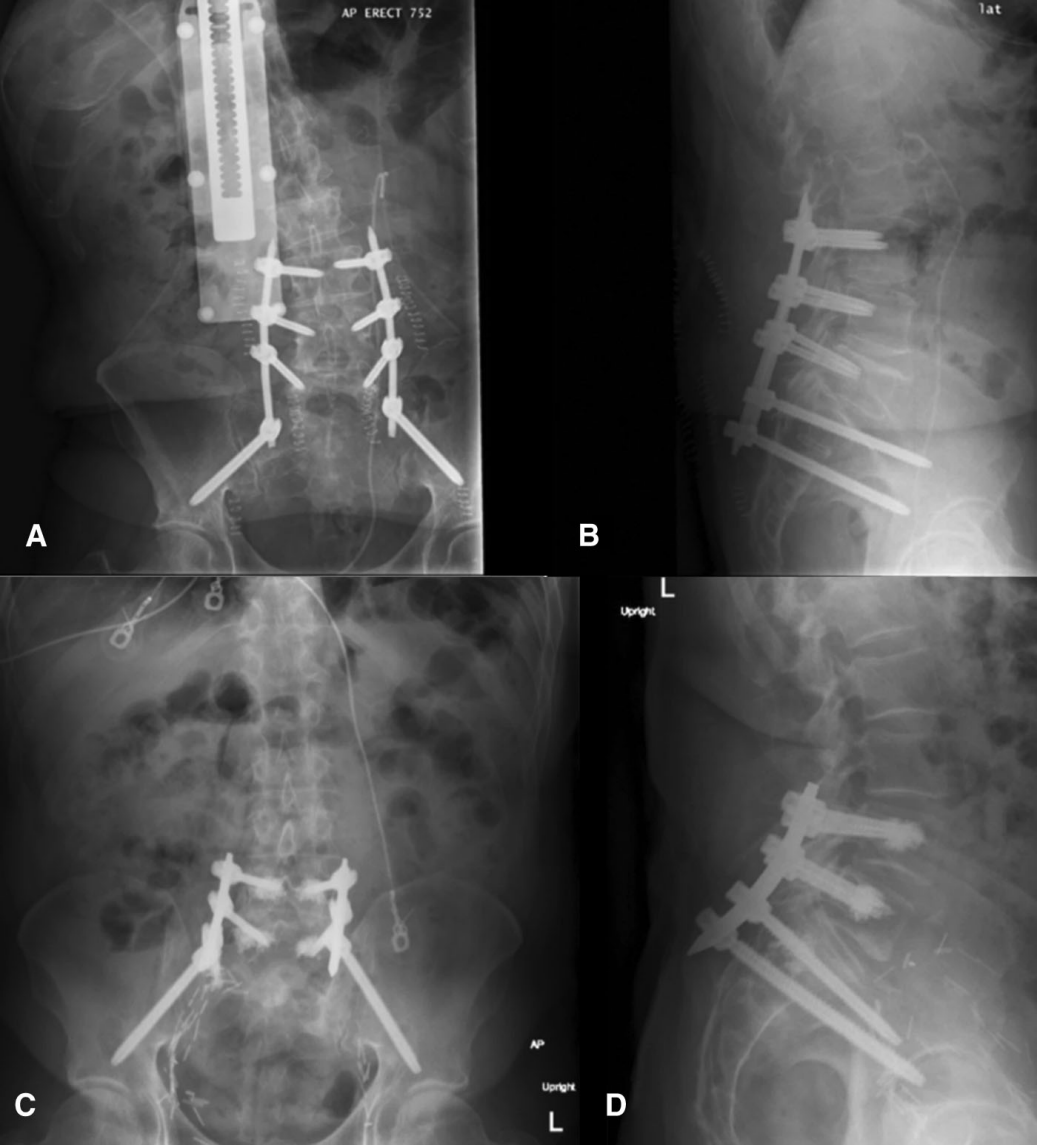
Postoperative upright x-rays for Cases 1 ((A) AP; (B) lateral) and 2 ((C) AP; (D) lateral) of the fixation construct demonstrating good screw placement (from (22), used with permission).
